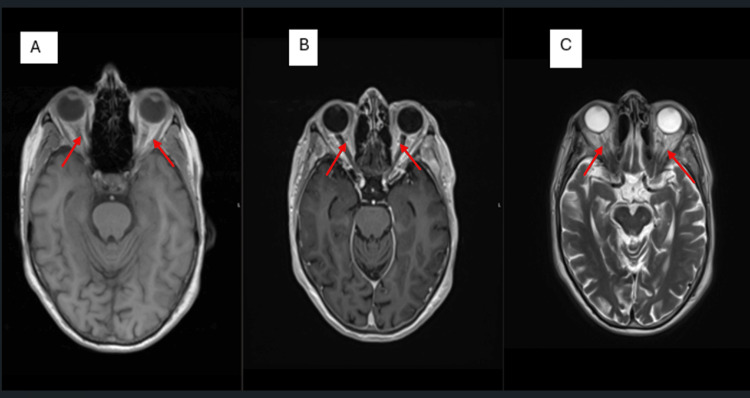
Magnetic resonance imaging (MRI) head showing bilateral optic nerve signal changes. A: precontrast MRI T1, B: postcontrast MRI T1 and C: MRI fluid-attenuated inversion recovery (FLAIR) T2
